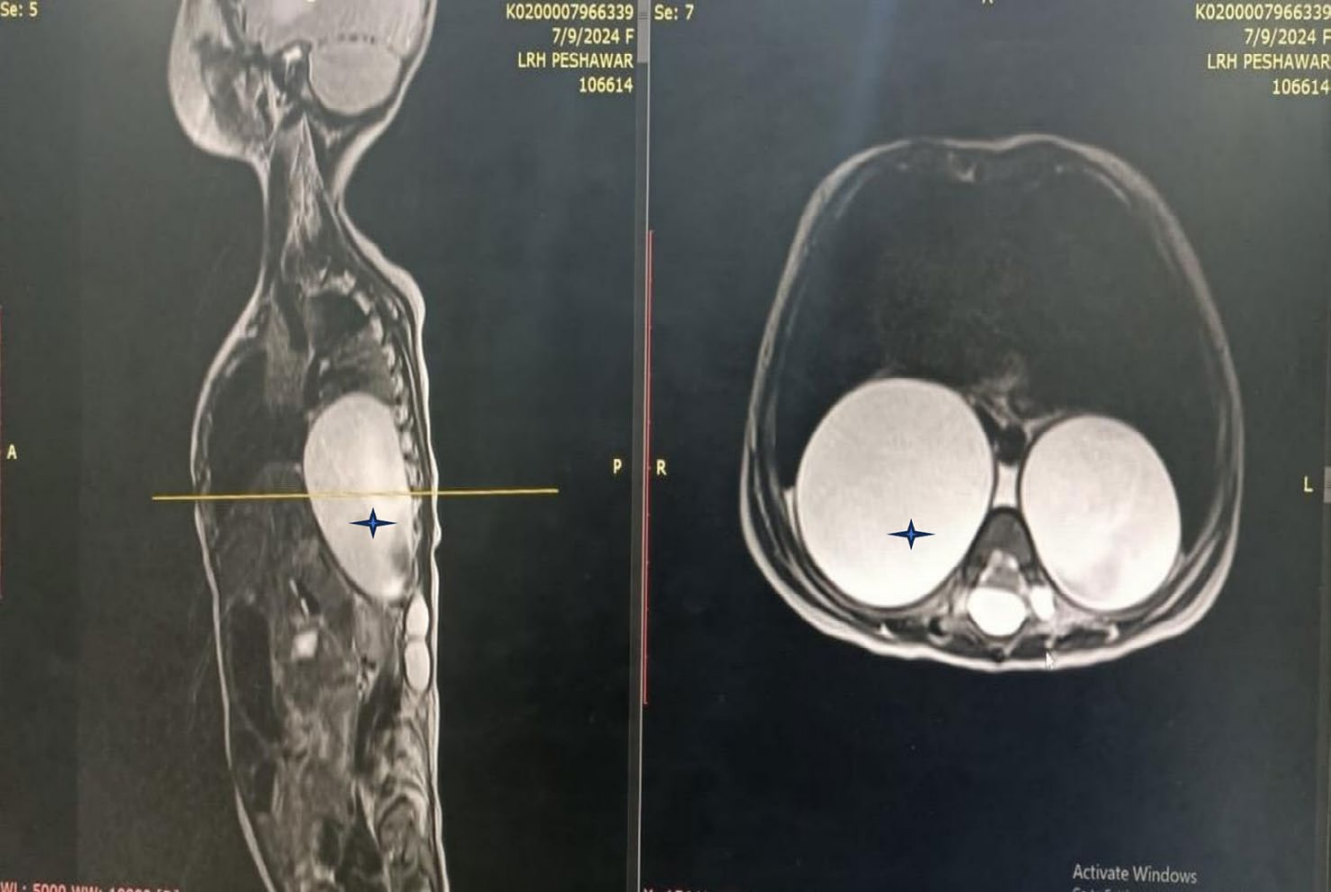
Magnetic resonance imaging (MRI) of the thoracic spine. These T2‐weighted images provide the definitive diagnosis of bilateral lateral meningoceles. The images show large cerebrospinal fluid (CSF)‐filled sacs (hyperintense signal, marked by asterisks) herniating through enlarged neural foramina. The absence of entrapped neural elements within the sacs is clearly demonstrated, confirming severe dural ectasia.
